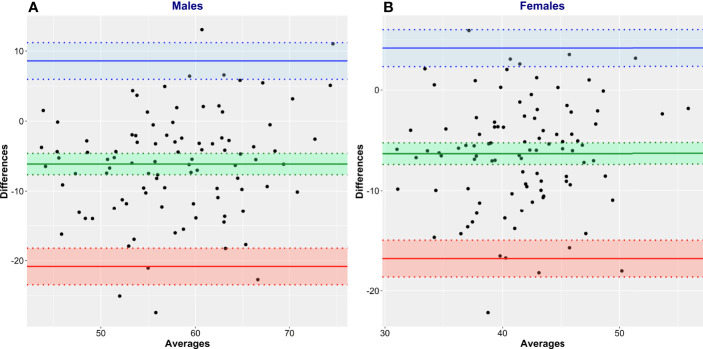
Bland and Altman plots of CT-defined lean body mass (LBM_CT_) vs. that estimated by James’ equation (LBM_James_). LBM_CT_ is the reference method, and LBM_James_ is the test method. Men (A) and women (B).
